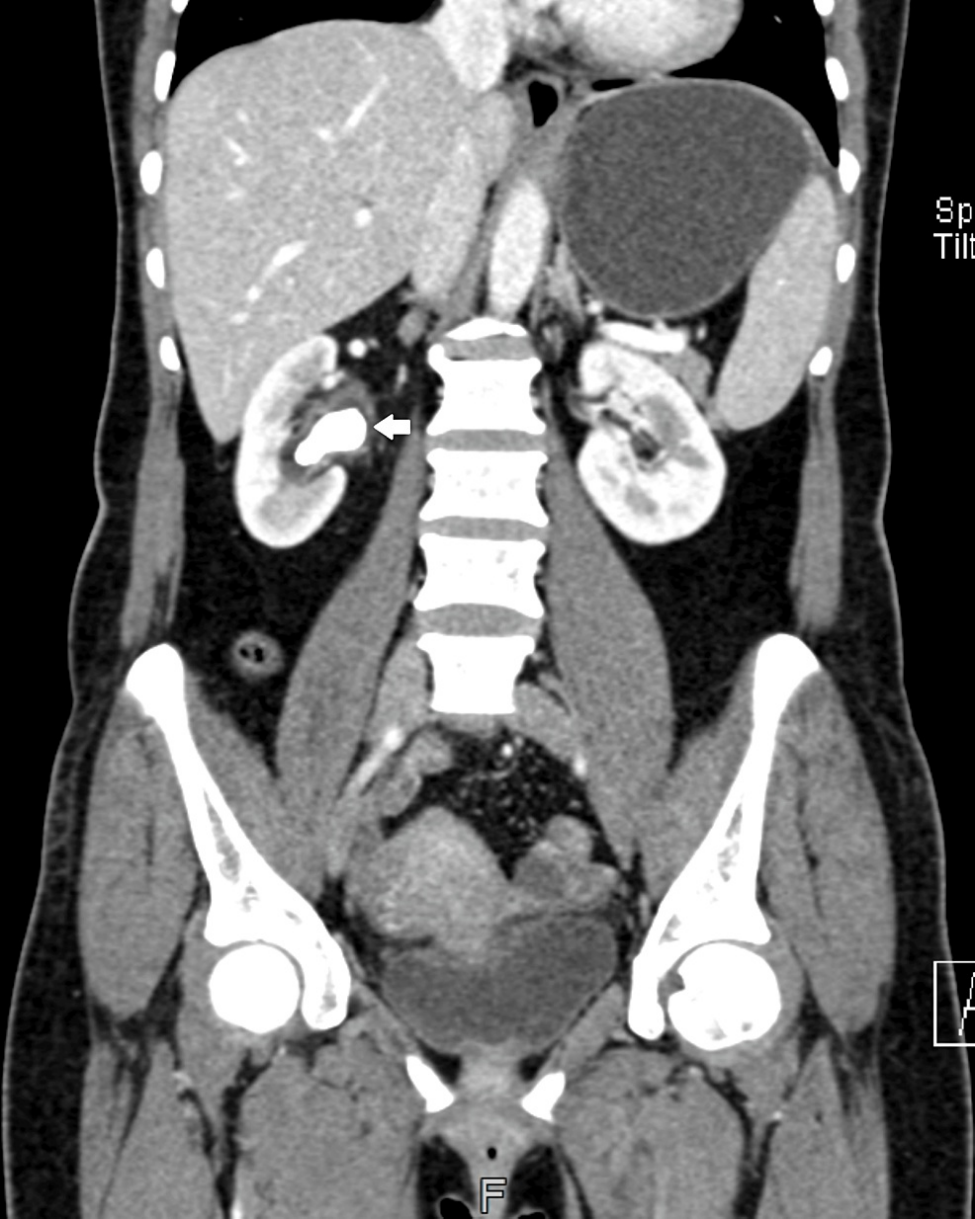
Contrast-enhanced computed tomography scan shows the right renal stone.
